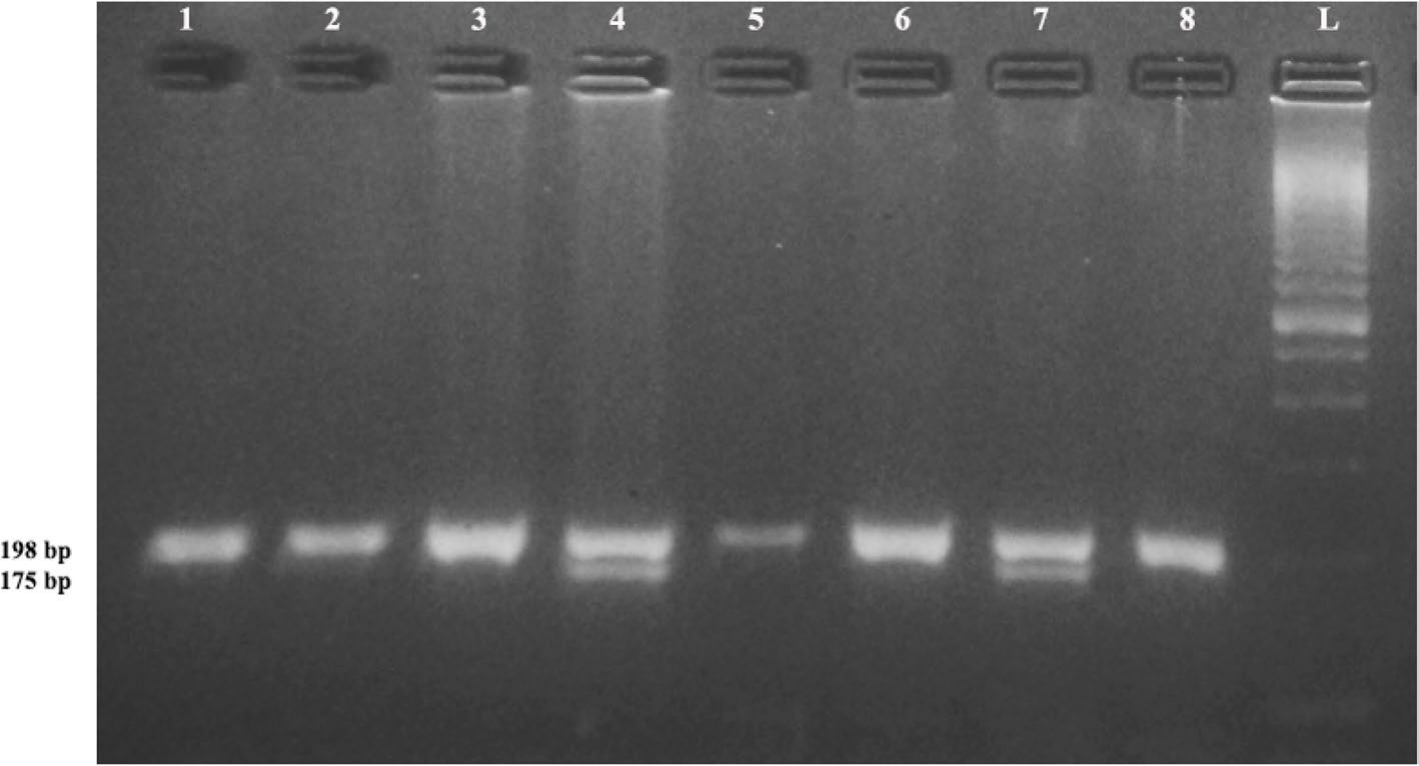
RFLP Gel Image of *MTHFR* C677T Polymorphism. L: 100 bp Ladder; Lane 1,2,3,5,6,8: 198 bp (CC: Wild); Lane 4,7: 198 + 175 + 23 bp (CT: Hetero). *23 bp band will not be visible on agarose gel
